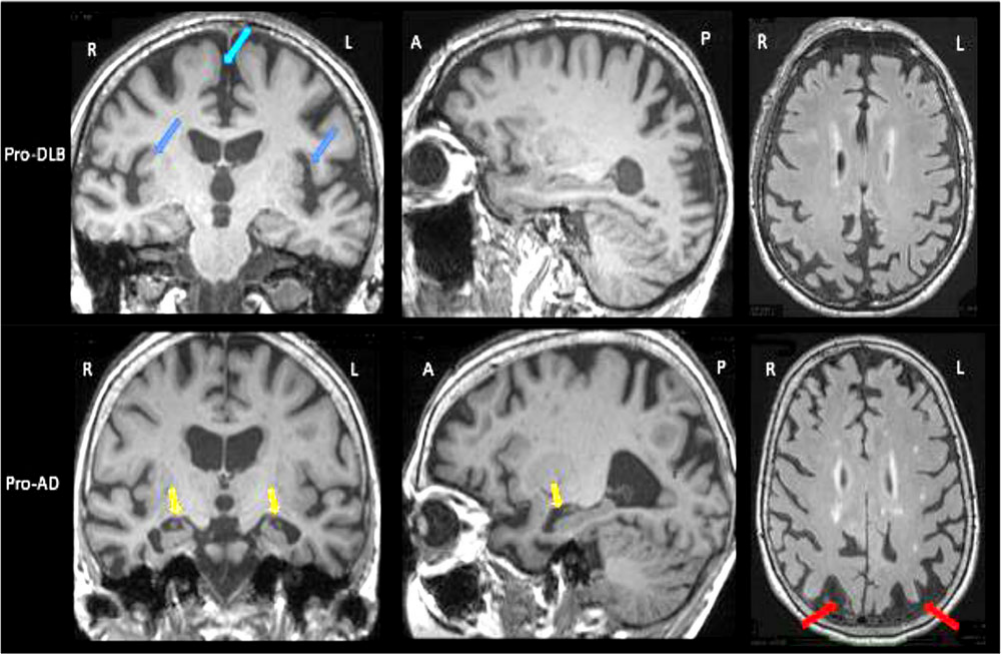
Biomarkers in MRI for AD.
